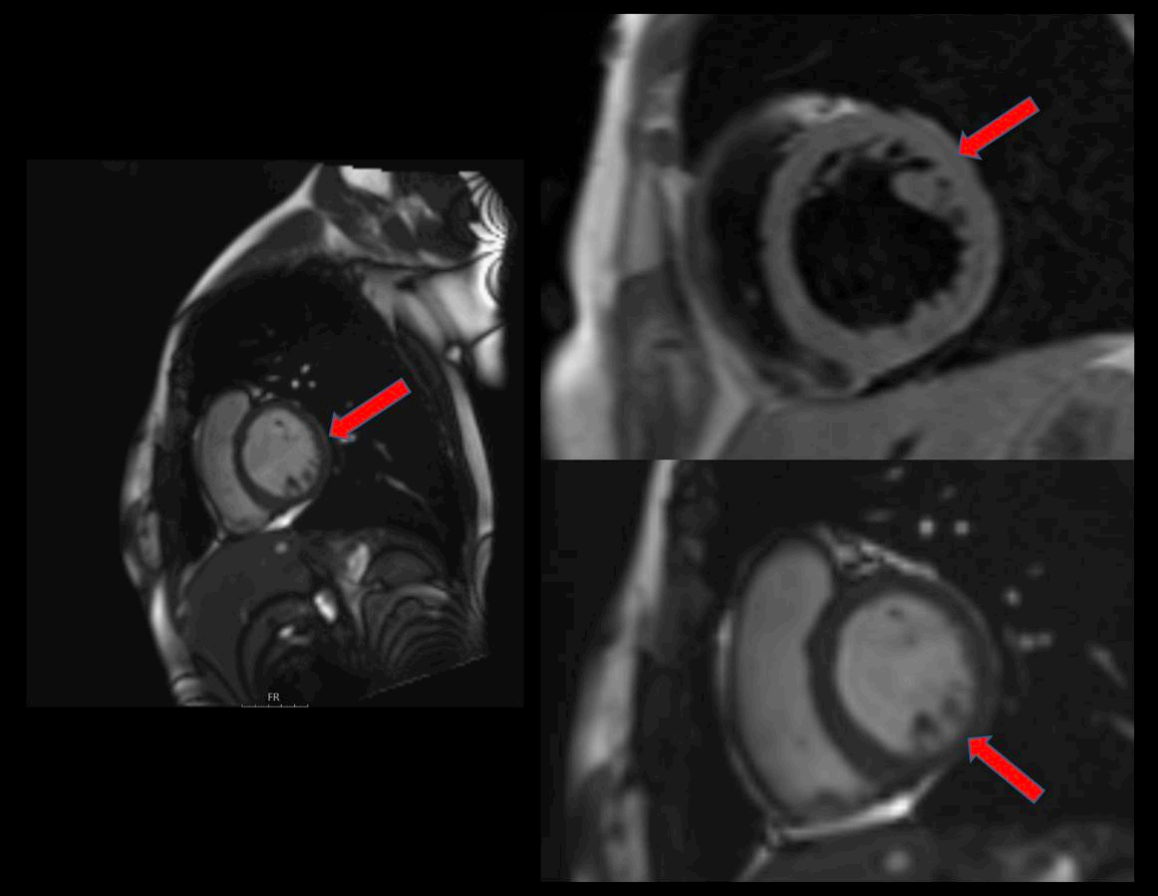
A cardiac magnetic resonance imaging showing the relationship of non-compacted to compacted myocardium up to 2.8 with enhanced trabeculations in the apical third of LV (arrows) with no signs of mural fibrosis.
